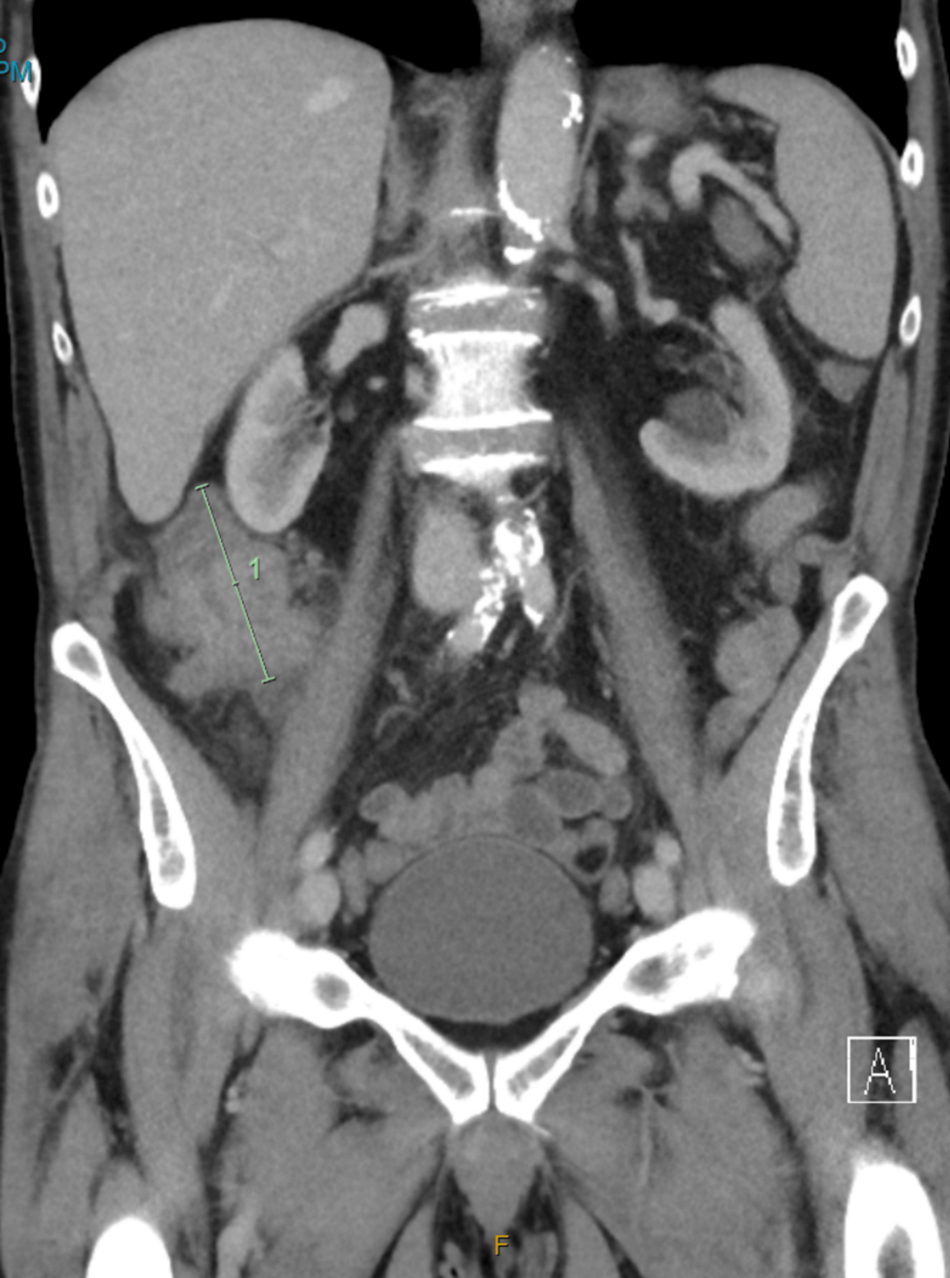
Coronal CT reconstruction indicating the tumor’s longitudinal extent. A green line indicating the location and length of the ascending colon tumor (1 unit length = 68 mm).
